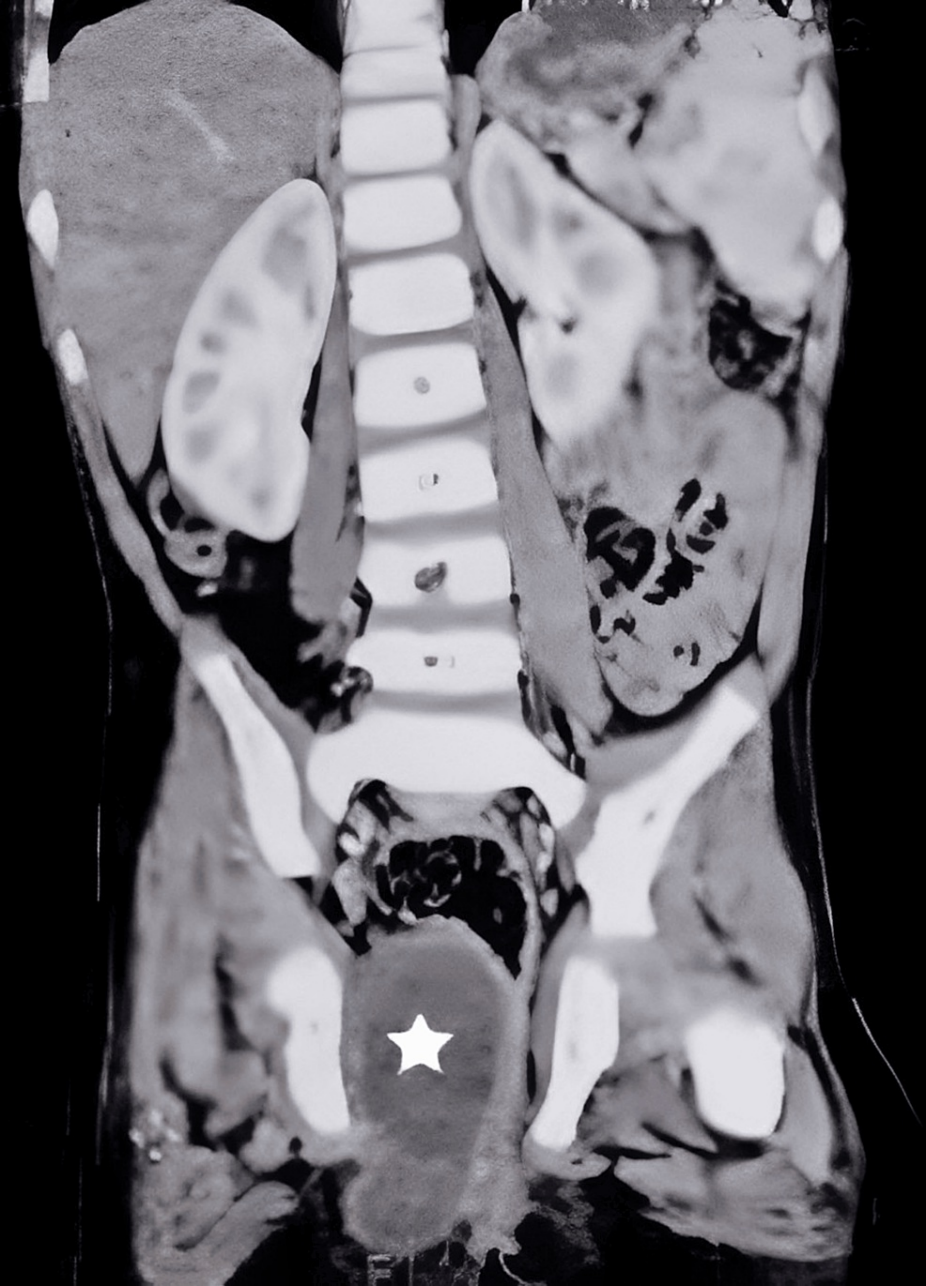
CT showing a 5x3 cm cystic lesion (asterisk) in the gluteal region compressing the anal canal.
